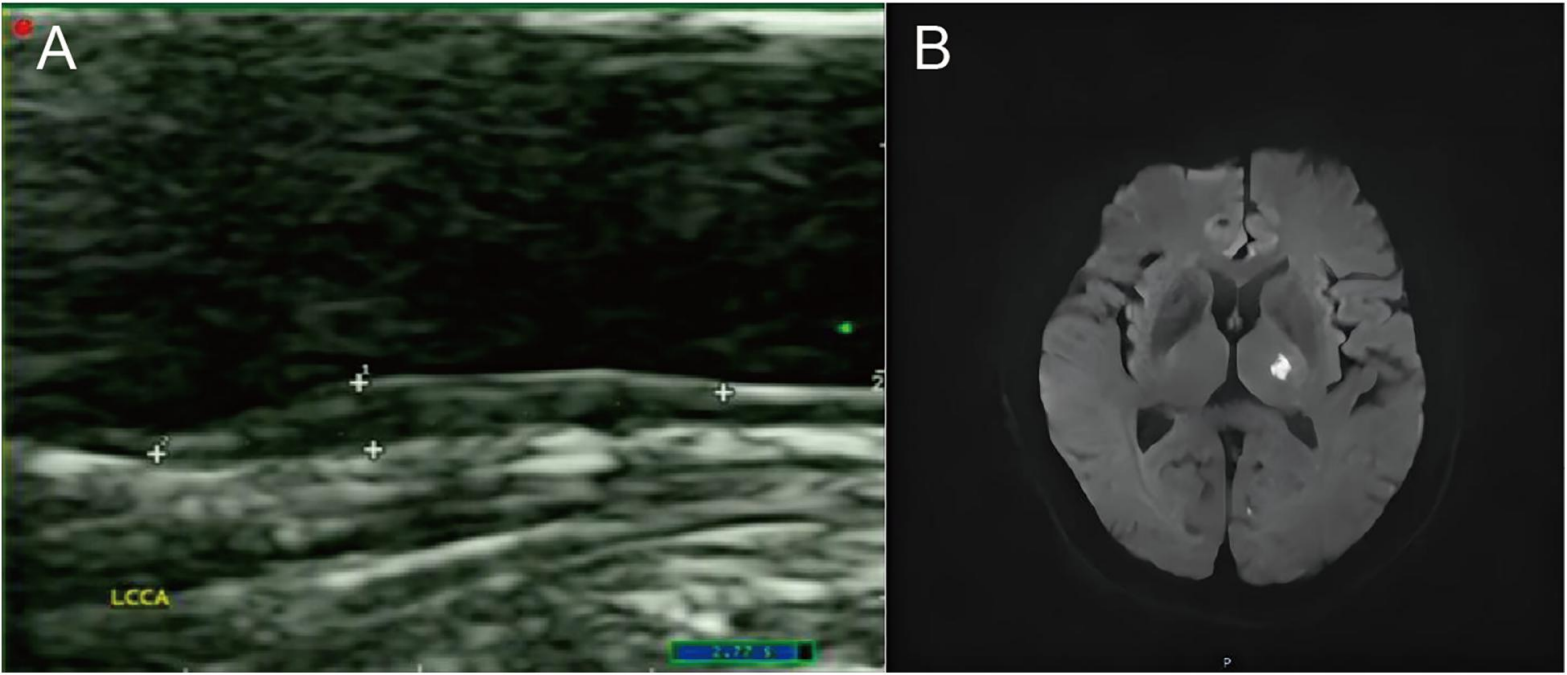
A case of an ACIS patient with a solitary Plaque-RADS-2 carotid plaque. (A) The sonogram shows a mainly hypoechoic plaque on the posterior wall of the left common carotid artery. (B) Diffusion-weighted imaging (DWI) sequence shows high signal in the left basal ganglia.
